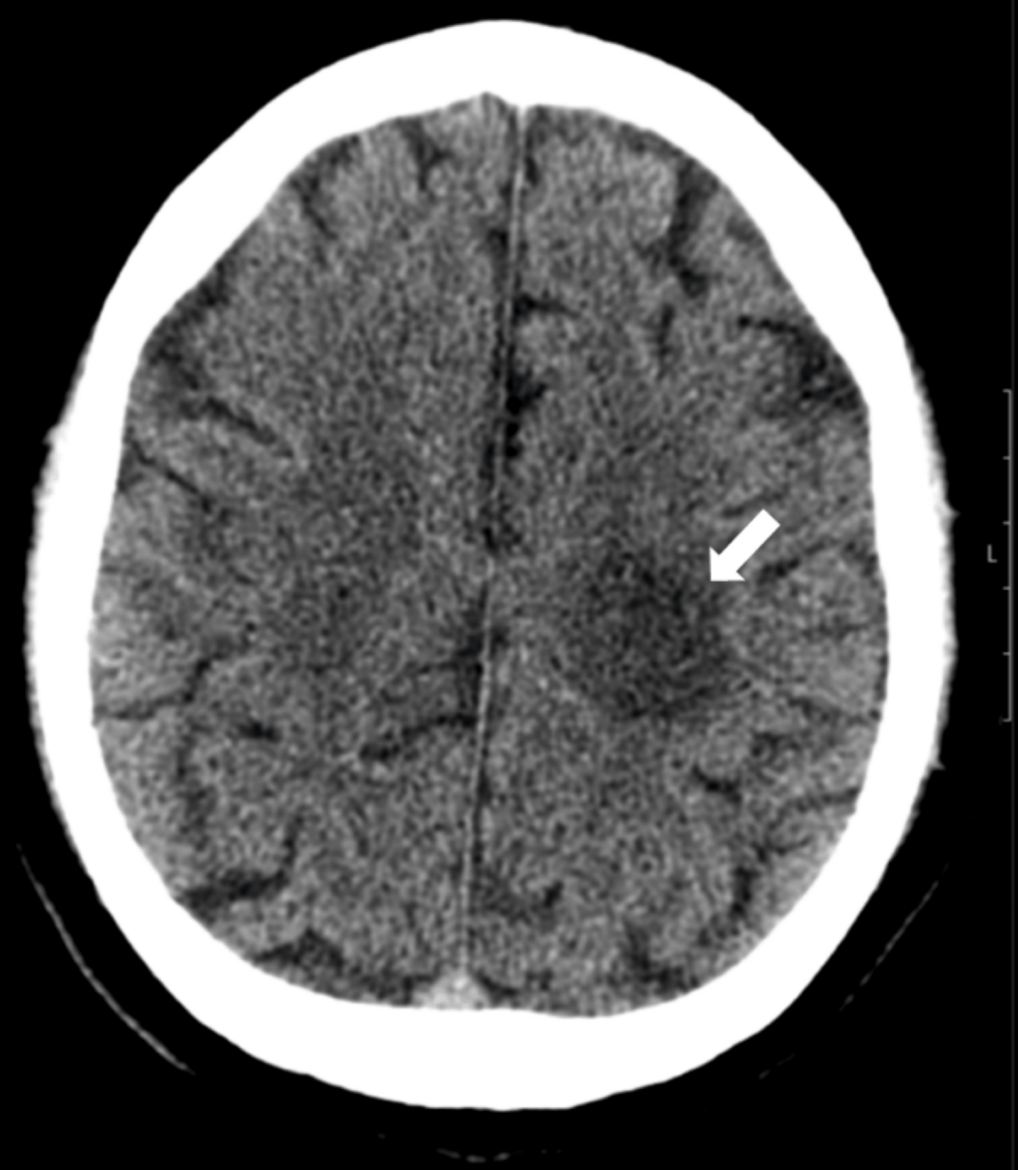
Non-contrast CT of the head. Axial slice shows a rounded hypodensity in the left subcortical superior parietal lobule (arrow), consistent with a focal lesion.
